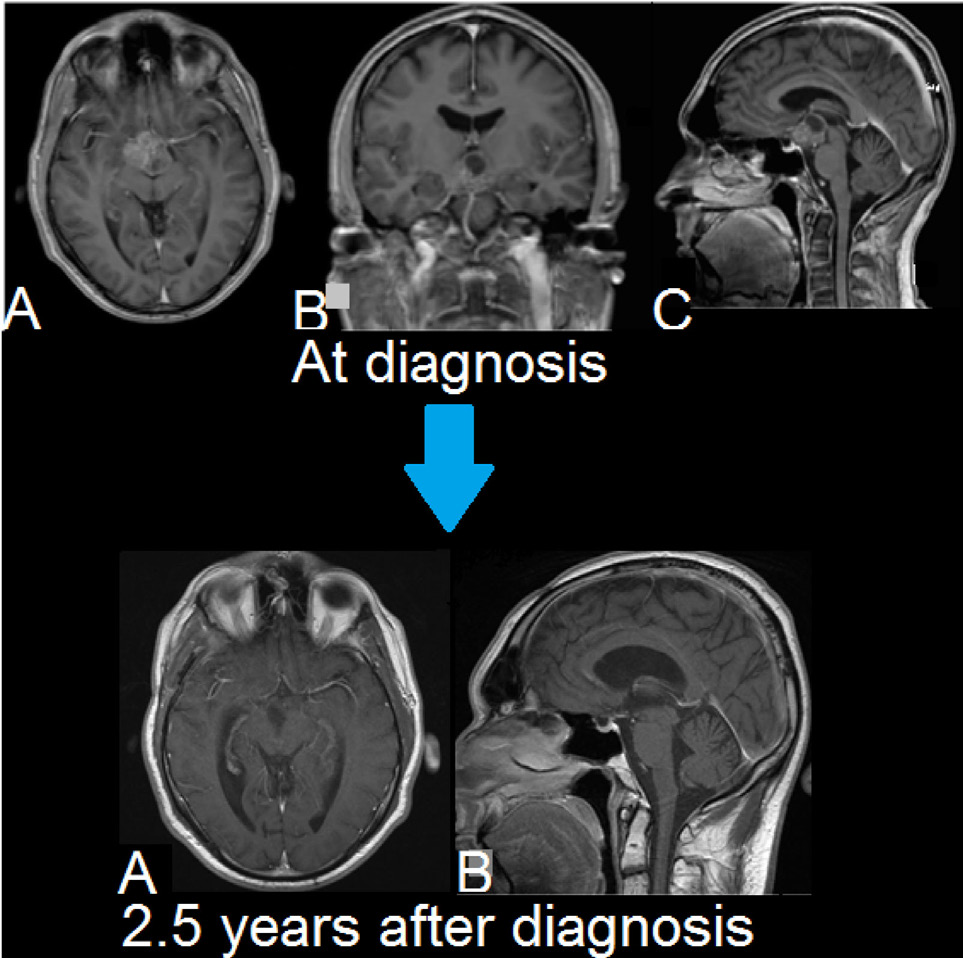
Second patient. MRIs of brain. Upper row (A–C): T1-weighted, Gd-enhanced (magnetization-prepared rapid gradient-echo (MPRAGE)). A suprasellar and interpeduncular lesion is seen with mixed solid and cystic (lobulated) elements. There is heterogeneous enhancement of intermediate intensity. Lower row (A,B): T1-weighted, Gd-enhanced. There is no evidence of tumor recurrence. Smooth linear enhancement is present at the resection site, which is consistent with the long-term changes seen following surgery.
